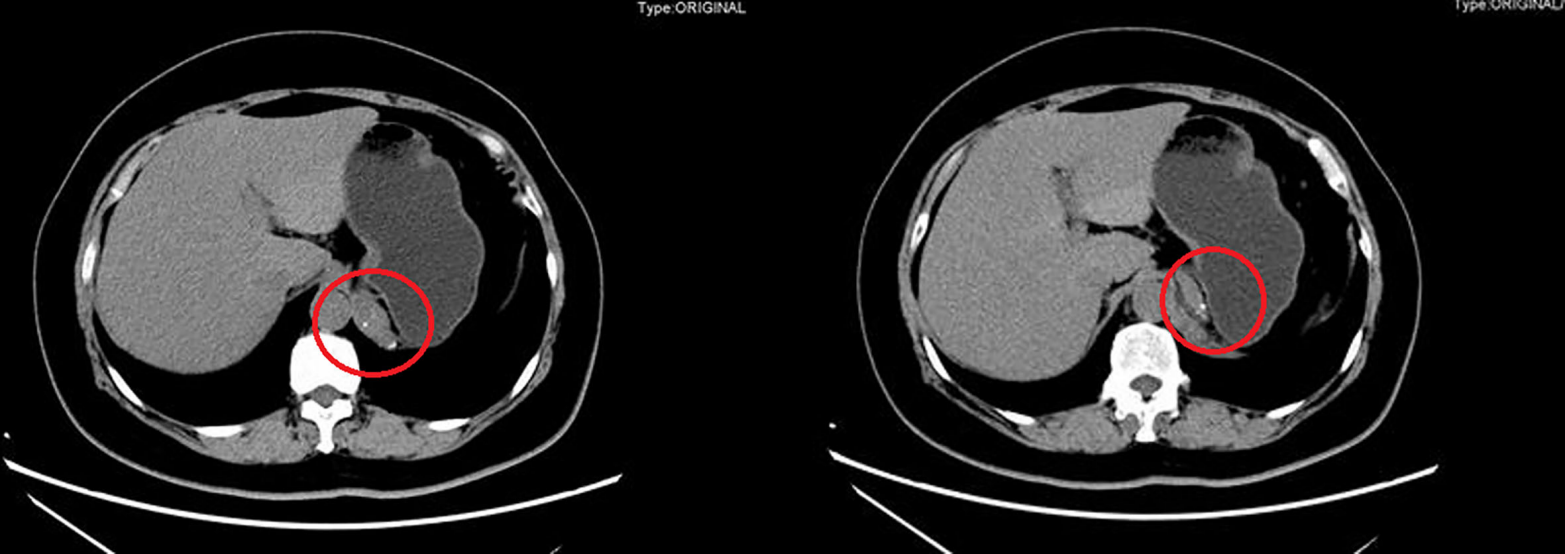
Computed tomography scan showed a 2.2×5.8 cm thin-walled water-attenuated cystic lesion in retroperitoneal region (red circle).
